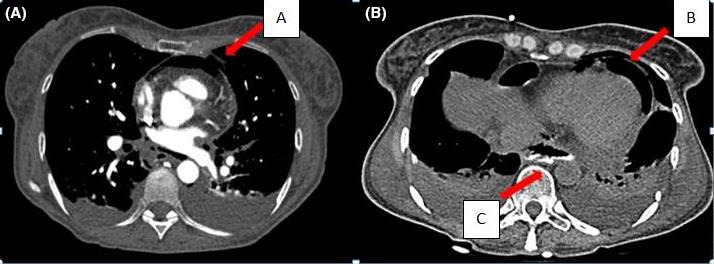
Chest CT scan with intravenous (A) and oral contrast medium (B) shows an idropneumopericardium (A, B arrows), pneumomediastinum, esophagopericardial fistula (C arrow), and bilateral pleural effusion.
